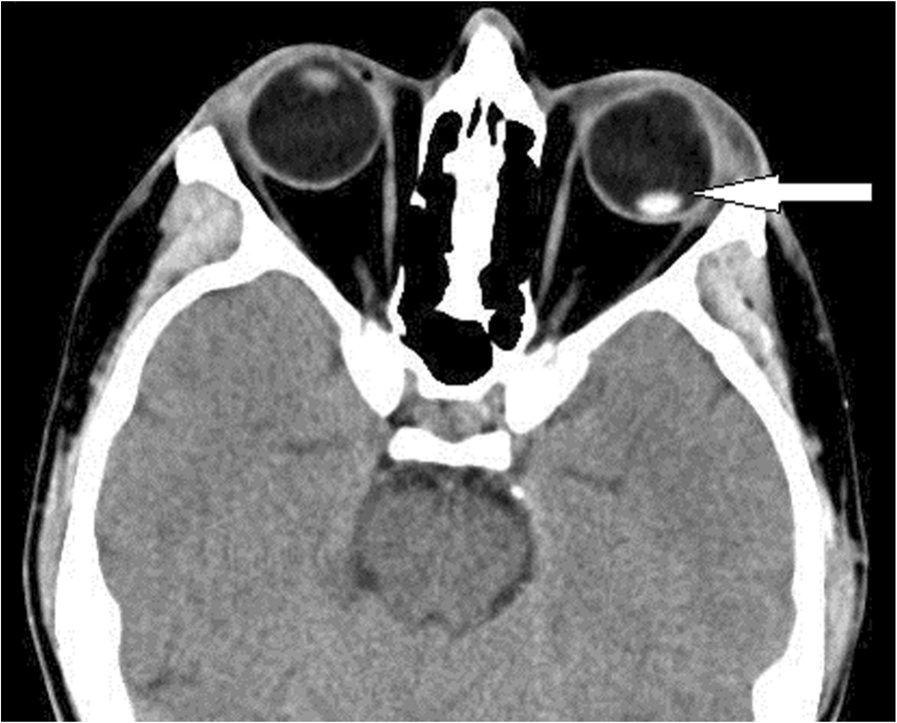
CT image corresponding to point-of-care ultrasound showing dislocated lens (*white arrow*)
